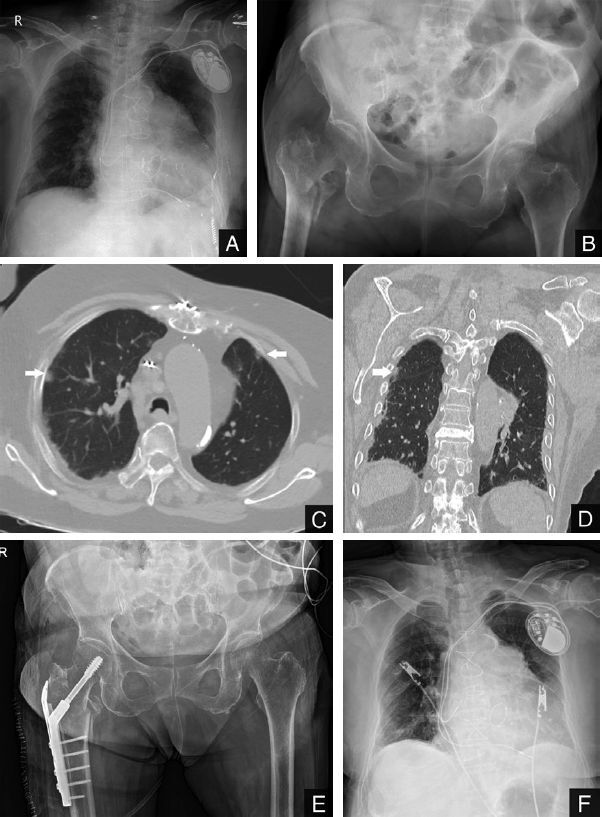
Radiographic images of the chest and the hip. Plain radiographs (Panels A and B) obtained at our emergency department show prominently increased interstitial opacities at the peripheral area in both lungs (A) and right femur intertrochanteric fracture (B). Axial and coronal CT images (Panels C and D) show nodular opacities based in the subpleural area (arrows) and a small amount of bilateral pleural effusion. Panels E and F are plain radiographs taken the day after surgery.
